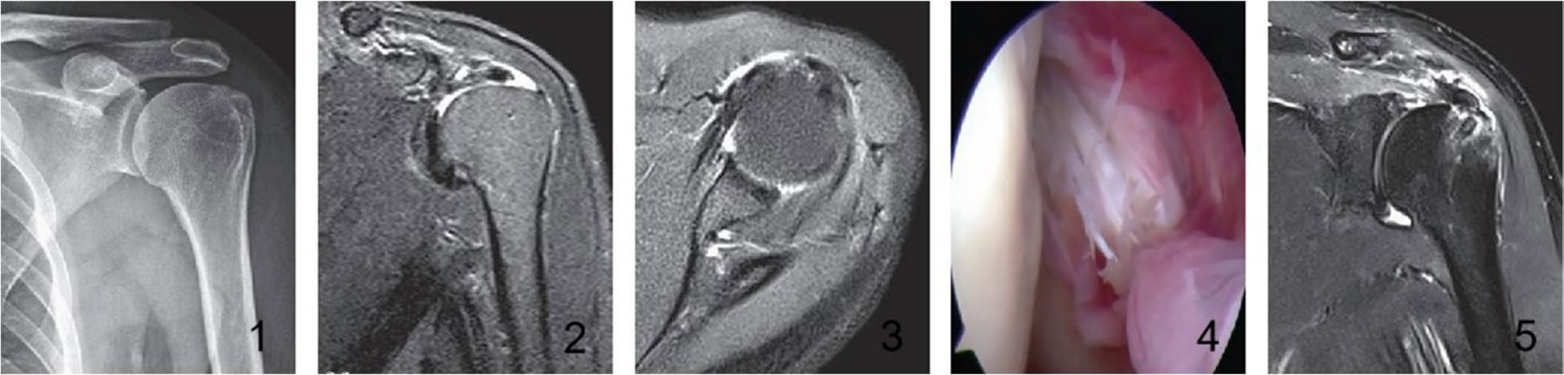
Note: 1–1: Preoperative anteroposterior radiograph shows an elevated acromion; 1–2: Preoperative oblique MRI T2WI of the left shoulder joint reveals a supraspinous tendon tear, Patte stage 2 retraction; 1–3: Preoperative transverse MRI T2WI of the left shoulder joint shows mixed hyperintensities; 1–4: Injury of the upper 1/3 of the subscapularis tendon (Lafoss I) was seen under the arthroscope intraoperatively, non-repaired; 1–5: MRI T2WI of the left shoulder joint at the final follow-up visit reveals an anchor in the greater tuberosity of the humerus and continuous recovery of the supraspinous tendon
